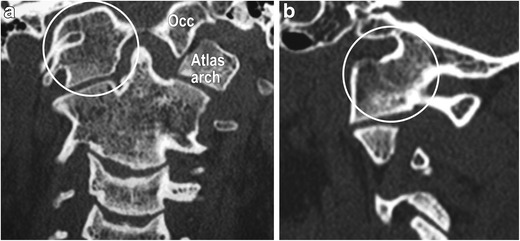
Atlanto-occipital assimilation in a 19-year-old female: Coronal (*a*) and sagittal (*b*) reformats from a cervical spine CT reveal abnormal fusion of the right-sided arch of atlas with the corresponding occipital condyle (*arrows*). The structures are clearly separate on the left
